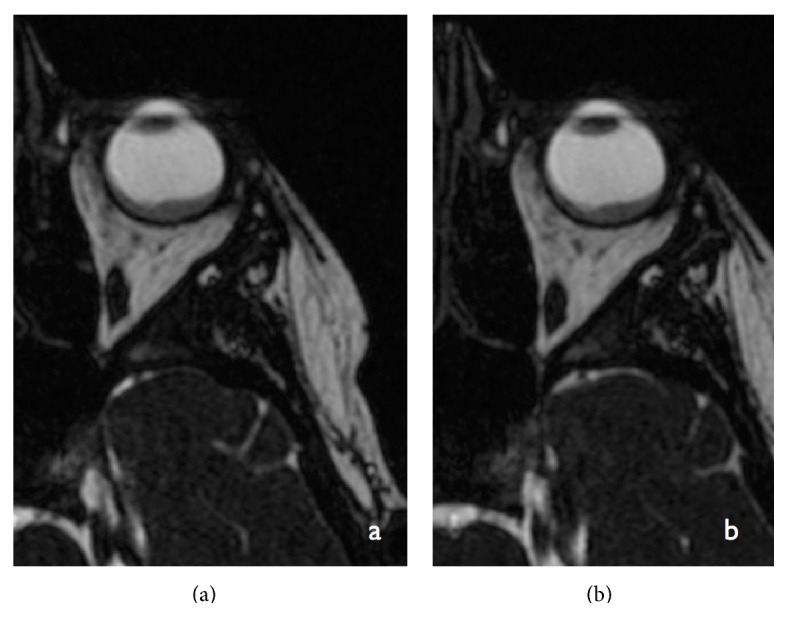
Axial CISS (constructive interference in steady state) MRI images showing that theretinal choroidal lesion shows similar signal characteristics as on T2-weighted images. No calcific signal foci are seen within the lesion. The CISS images provide high spatial resolution and help in radiologically defining the extent of the tumor.
